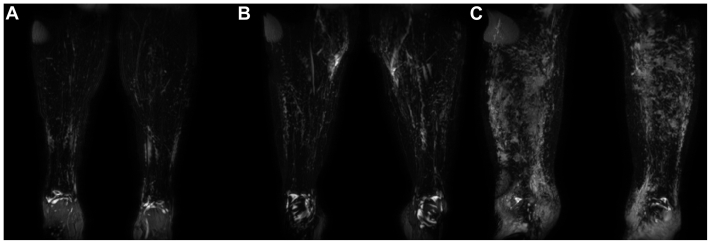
Examples demonstrating a range of T2-weighted images from controls and lymphedema patients. Single slices of heavily T2-weighted images of the mid-leg for 31- (A), 37- (B), and 27- (C) year-old women with identical image windowing and obtained using the same imaging protocol. The 37-year-old healthy control participant showed the most extensive hypointense regions in T2-weighted images of any control included in this study (B). C, A T2-weighted scan from a similarly aged lymphedema patient (diagnosed with WILD [warts, immunodeficiency, and lymphatic dysplasia] syndrome^20^), with extensive high-signal, fluid-rich, regions throughout the entire limb. B, Based purely on a physical inspection of the limbs, the clinician performing the contrast injection suggested that an undiagnosed lipedema could be present, which might explain the increased fluid signal.
